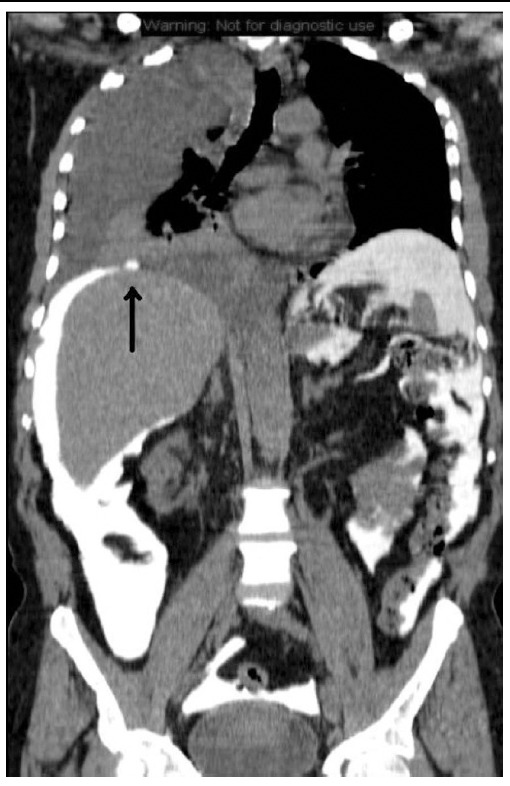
Computed tomography of the thorax shows small contrast filled bleb like lesion in right posterior diaphragm (arrowed).
